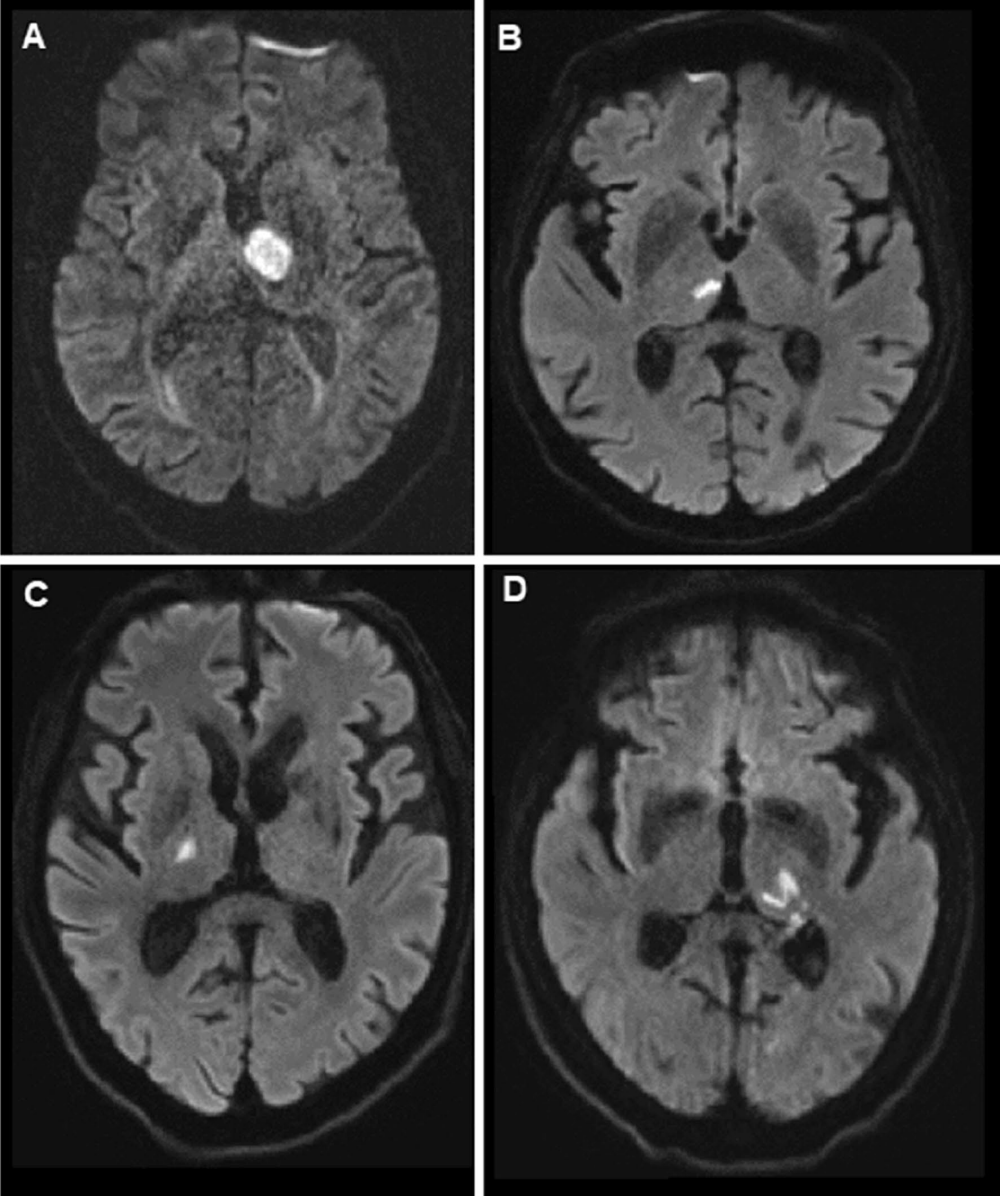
Examples of IALT localizations in the DWI sequence: A left anterior B right paramedian C right inferolateral D left posterior
